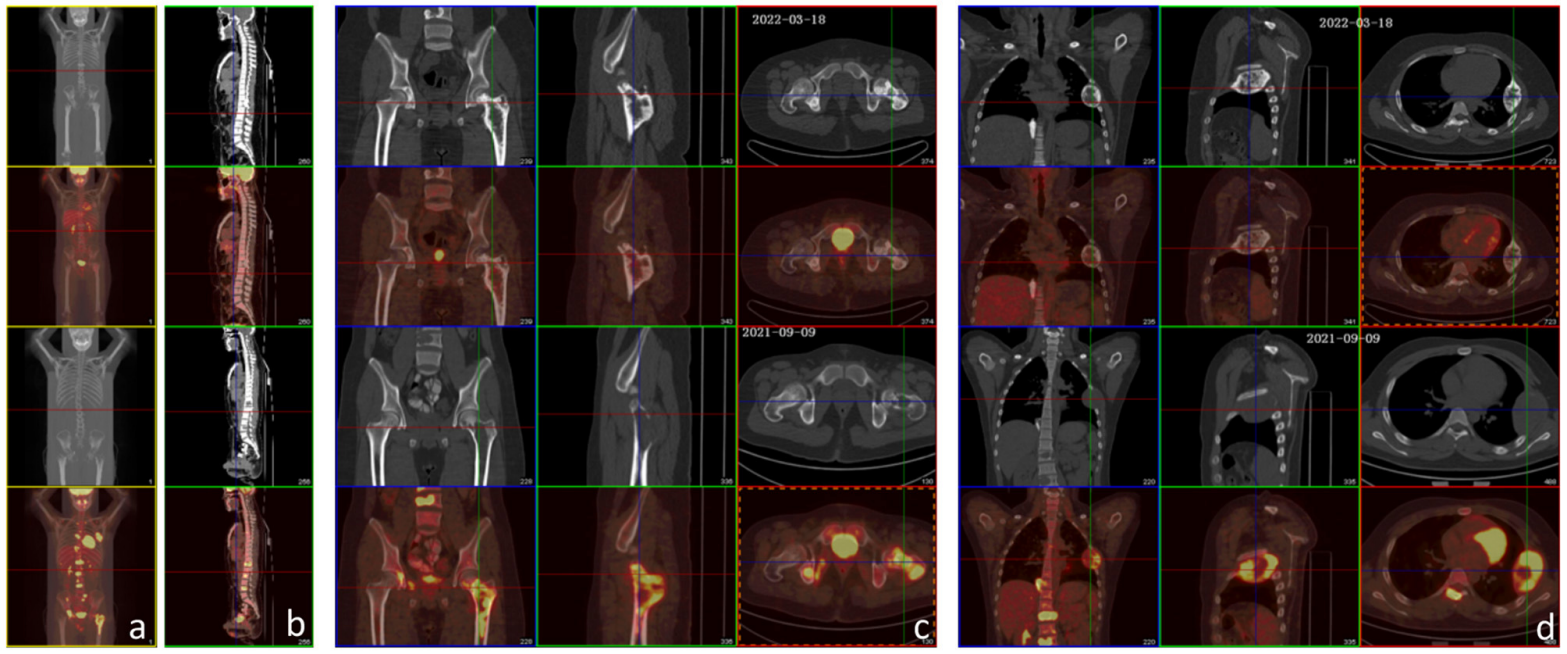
PET-CT examination before treatment and after the 6th chemotherapy. (a–d) The lower two rows are images before treatment, and the upper two rows are images after the 6th chemotherapy. After treatment, FDG metabolism in all metastases decreased (bilateral paraacromial side of the clavicle, left 5th and 6th posterior intercostal space, right lower lobe of the lung, lymph nodes in mesentery area, and multiple parts of the whole body: T2–T12, L1–L4 vertebral bodies, T2, T6, T12, and L2 attachments, left 4th rib, right 11th posterior rib and 8th anterior rib, left clavicle, sacrum, femoral head and upper segment of the femur, right acetabulum, and sciatic bone), except that the local FDG metabolism at the proximal left femur was still higher than normal (significantly lower than that before treatment). The multiple bone metastases showed varying extents of high-density calcification. There was little change from the mastoid process to the petrous tip of the right temporal bone, showing irregular morphology and increased bone density, and no significant increase in FDG metabolism.
